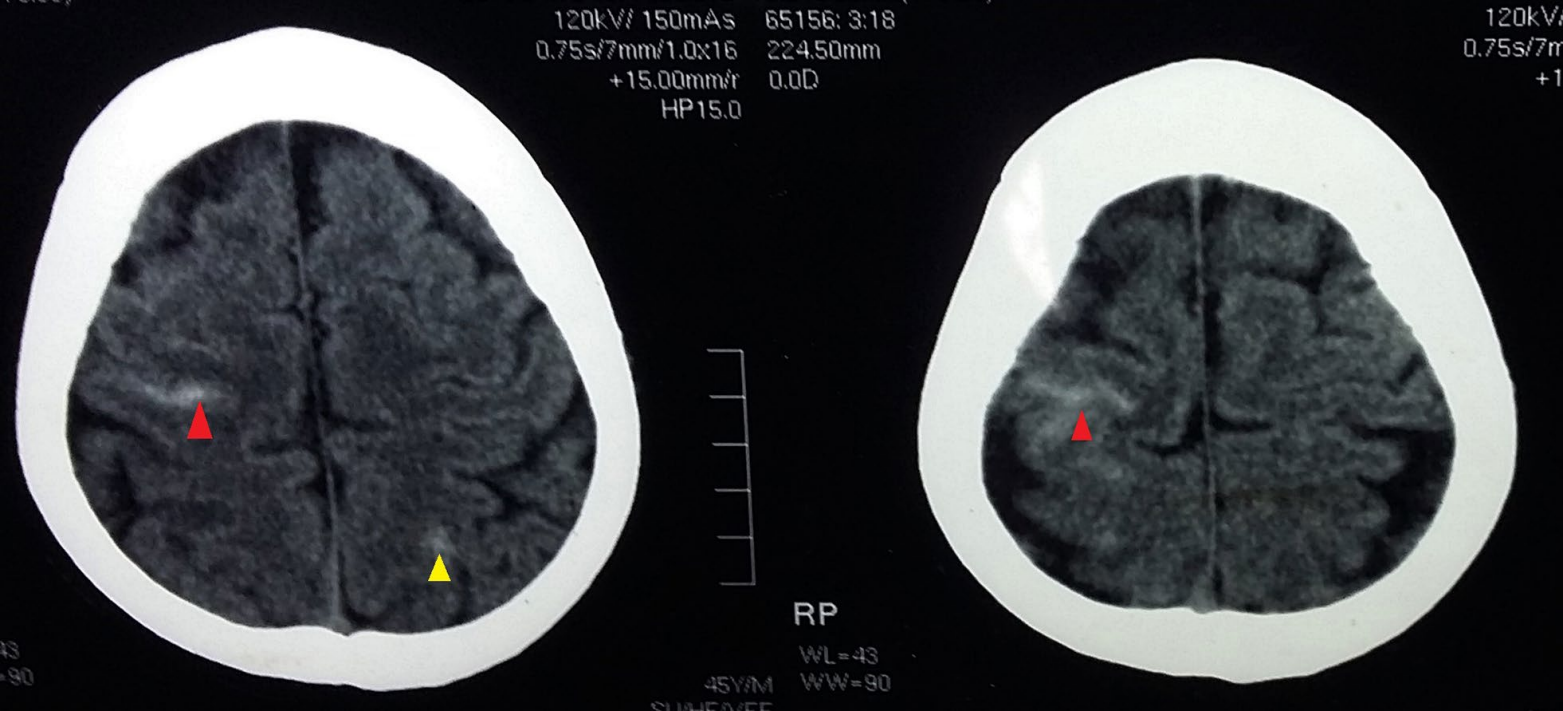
Computed tomography (CT) scan of the head: showing subarachnoid (red) and intracranial haemorrhages (yellow)
